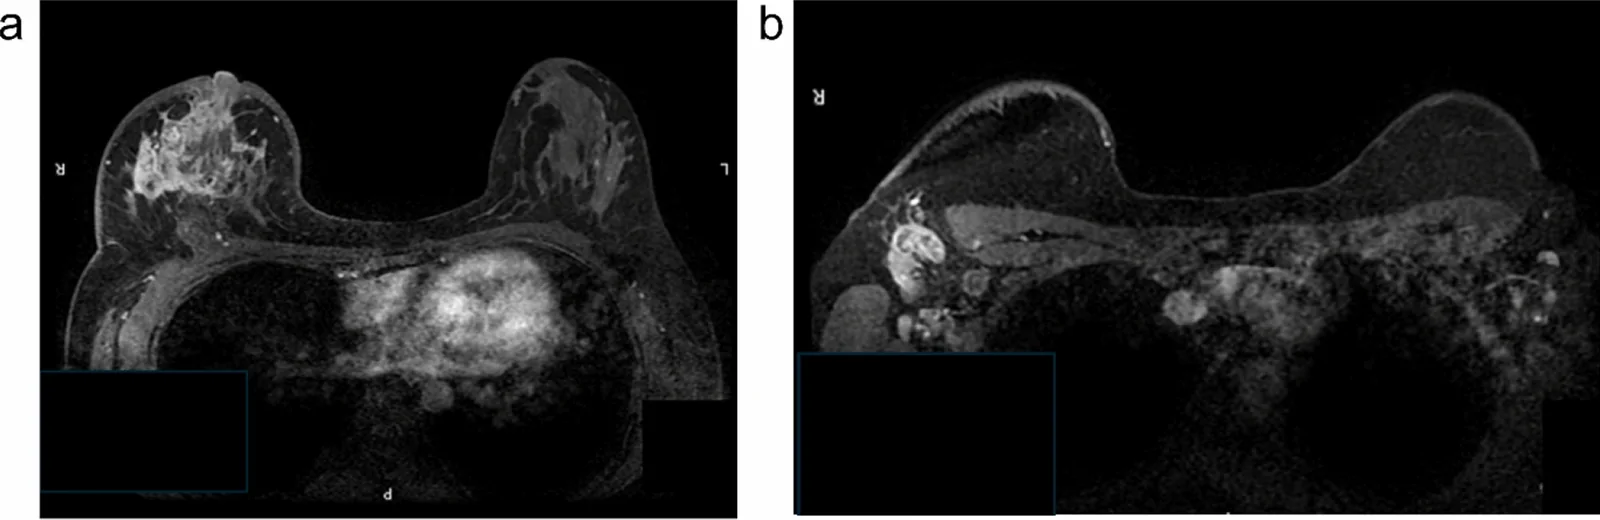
Contrast-enhanced breast magnetic resonance imaging (MRI) findings before treatment initiation.(a) Contrast-enhanced breast MRI demonstrating an extensive enhancing lesion in the right breast; (b) Contrast-enhanced MRI showing enlarged right axillary lymph nodes suspicious for metastasis
